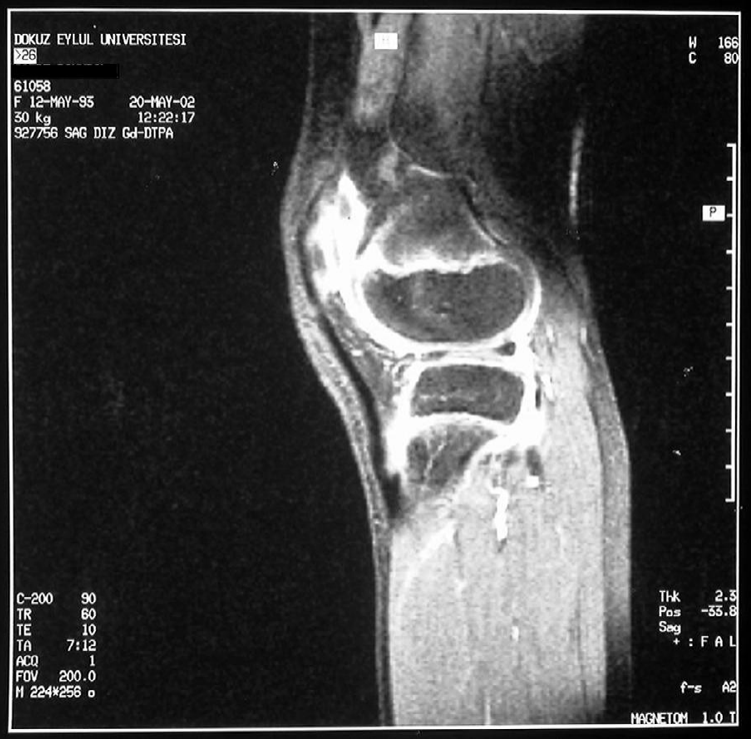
Contrast enhanced MRI of the right knee demonstrating chronic inflammation with synovial thickening.
